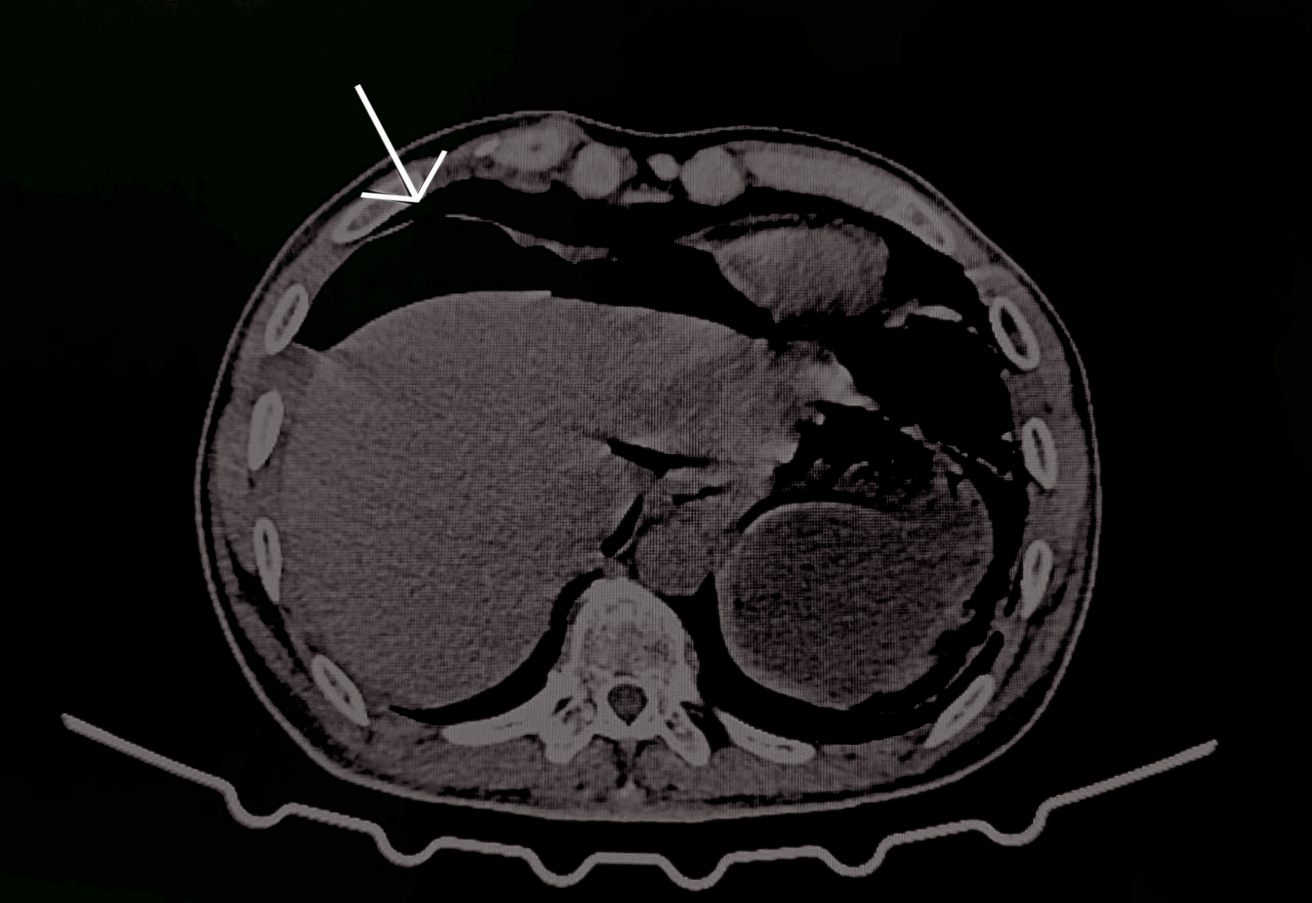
CT image showing D1 perforation (marked with white arrow) CT, computed tomography; D1, duodenum 1
